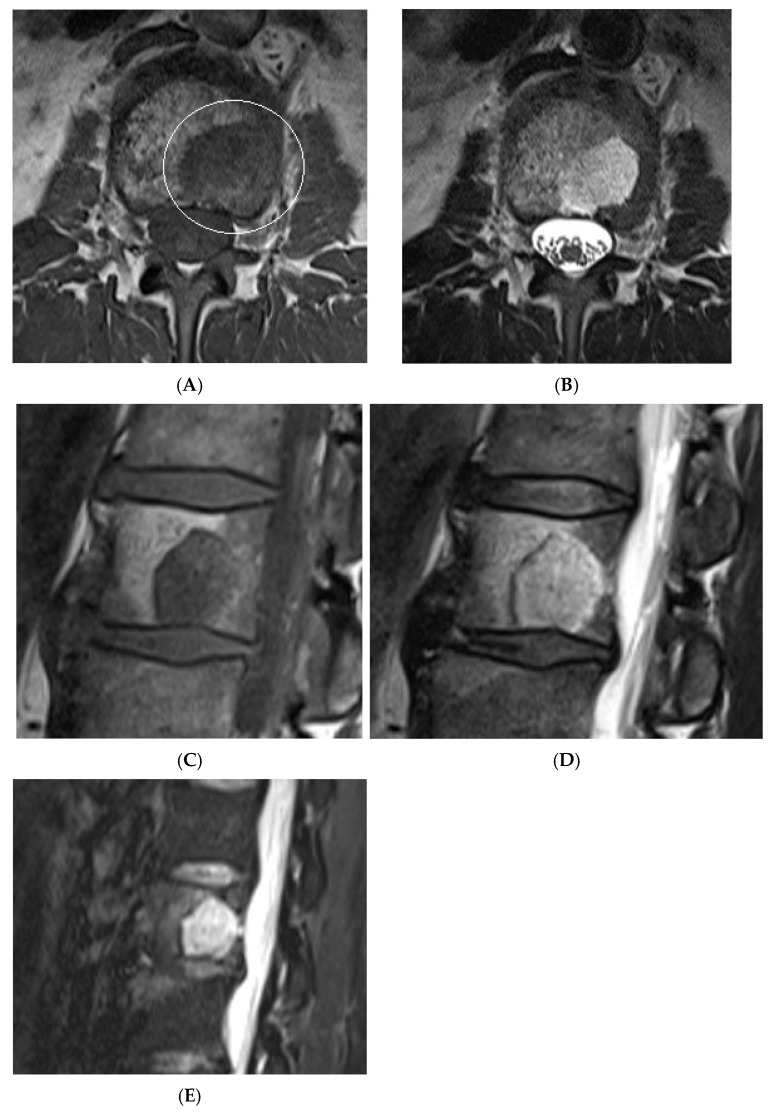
Collision Lesions: Typical and atypical haemangioma of vertebral body. (A) Axial T1W MRI demonstrating a classic haemangioma with a T1 hyperintense lesion occupying the vertebral body, with a smaller atypical T1 hypointense lesion (circled) adjacent, in keeping with an atypical haemangioma. (B) Axial T2W MRI with the same lesions—the atypical lesion to the left side of the patient (vertebral body) has a higher vascular component and therefore is more hyperintense than the adjacent and larger typical haemangioma. The larger lesion still remains hyperintense on T2, in keeping with predominant internal fat content. (C) Sagittal T1W spine MRI. (D) Sagittal T2W spine MRI. (E) Sagittal STIR spine MRI demonstrating fat suppression within the typical haemangioma with hyperintense signal within the atypical haemangioma as a collision lesion example.
